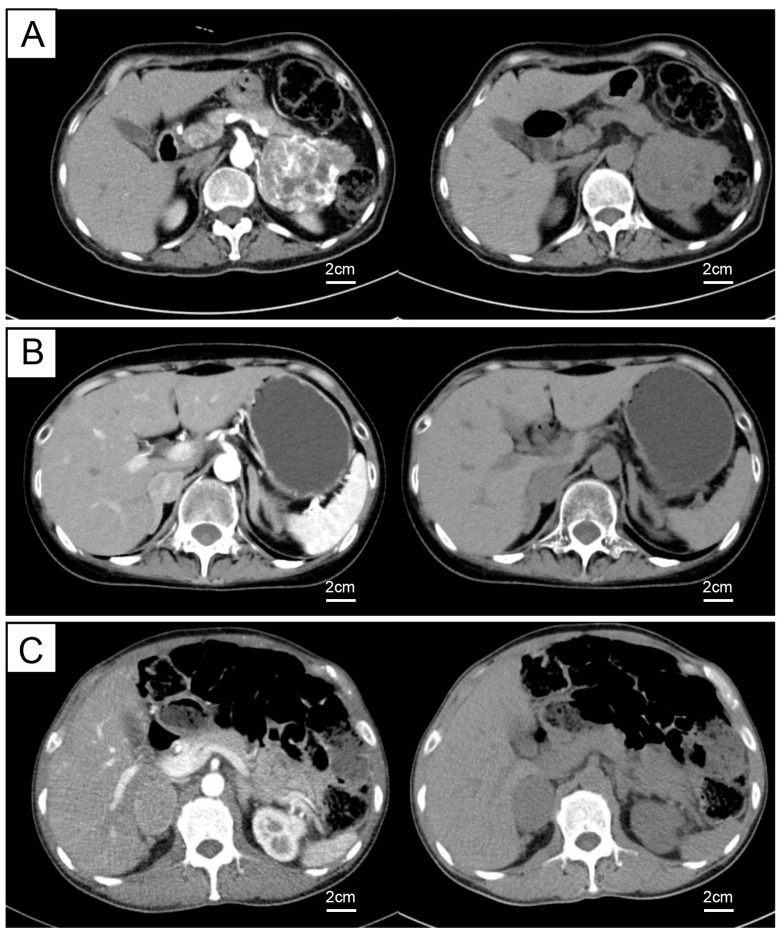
Enhanced CT characteristics of eas-PCC in three cases. (A–C) Enhanced CT images of eas-PCC in three cases, shown in the arterial phase and unenhanced phase. Scale bar = 2 cm.
